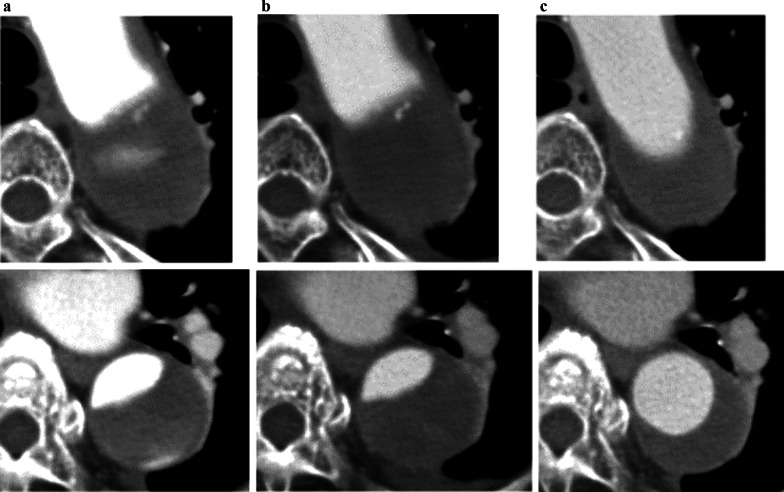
Preoperative and postoperative computed tomography. a Blood flow is observed in the false lumen in preoperative CT. b In an early postoperative CT, blood flow has disappeared from the false lumen. c In a CT 1-year postoperatively, the diameter of the vessel has reduced, and the true lumen has increased in size
